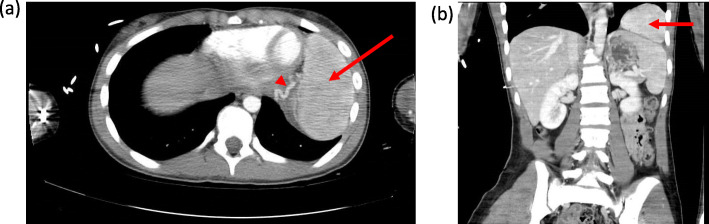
Transverse (a) and frontal (b) CT scan views of left chest mass in our 14 year old patient. Arrows pointing at the intrathoracic accessory spleen. Arrow head showing inferior phrenic artery blood supply
